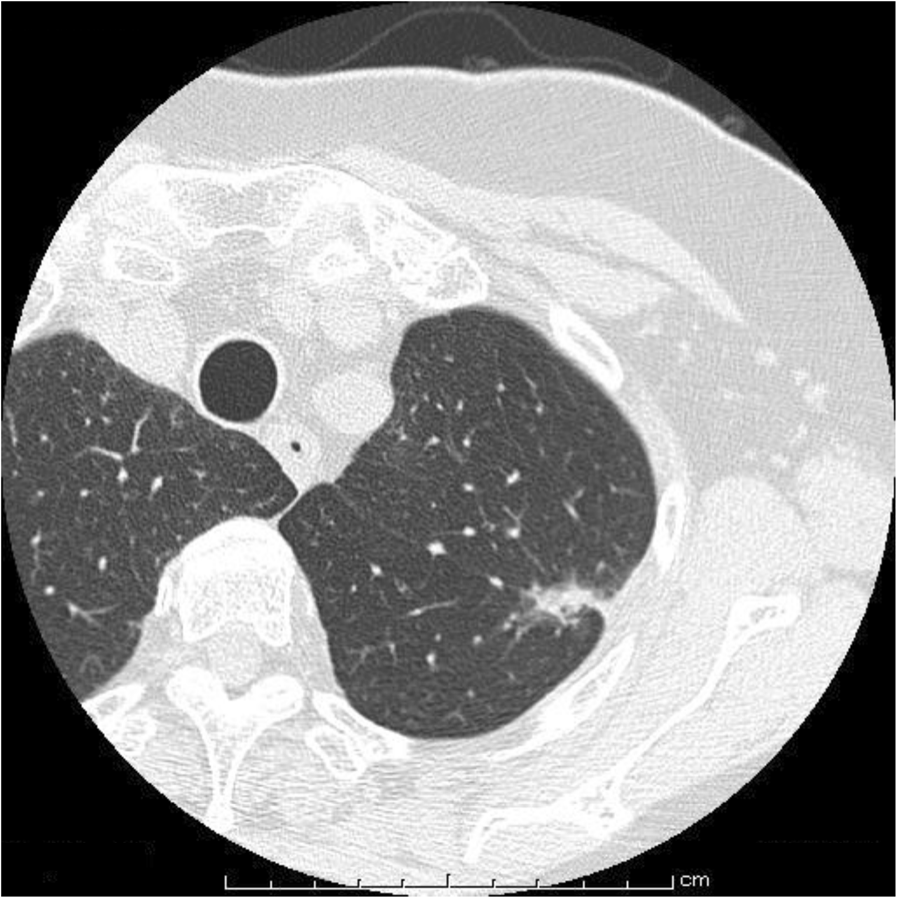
Chest-computed tomography findings. It shows the nodule of left S1+2 with indentation. It is 22 mm in size
